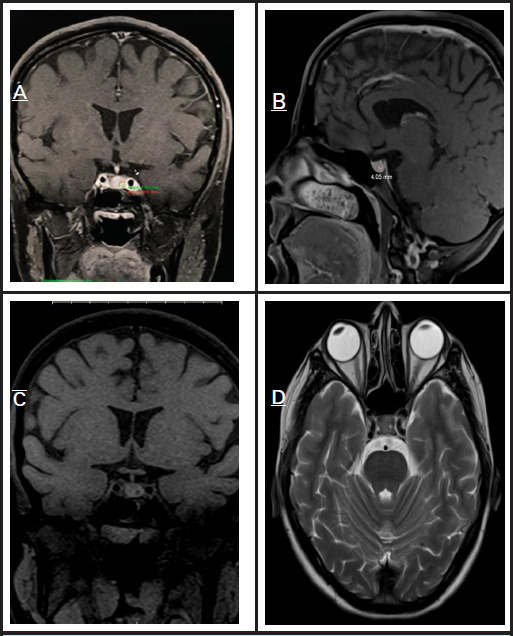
MRI images of the pituitary gland demonstrate a single nodule consistent with a pituitary adenoma located on the left-posterior aspect. (A) Coronal view showing the nodule on the left- posterior side of the pituitary gland. (B) Sagittal view revealing the same lesion measuring approximately 4 x 4 mm. (C) Coronal view confirming the localization of the nodule. (D) Axial view further supports the presence of the lesion on the left-posterior aspect.
